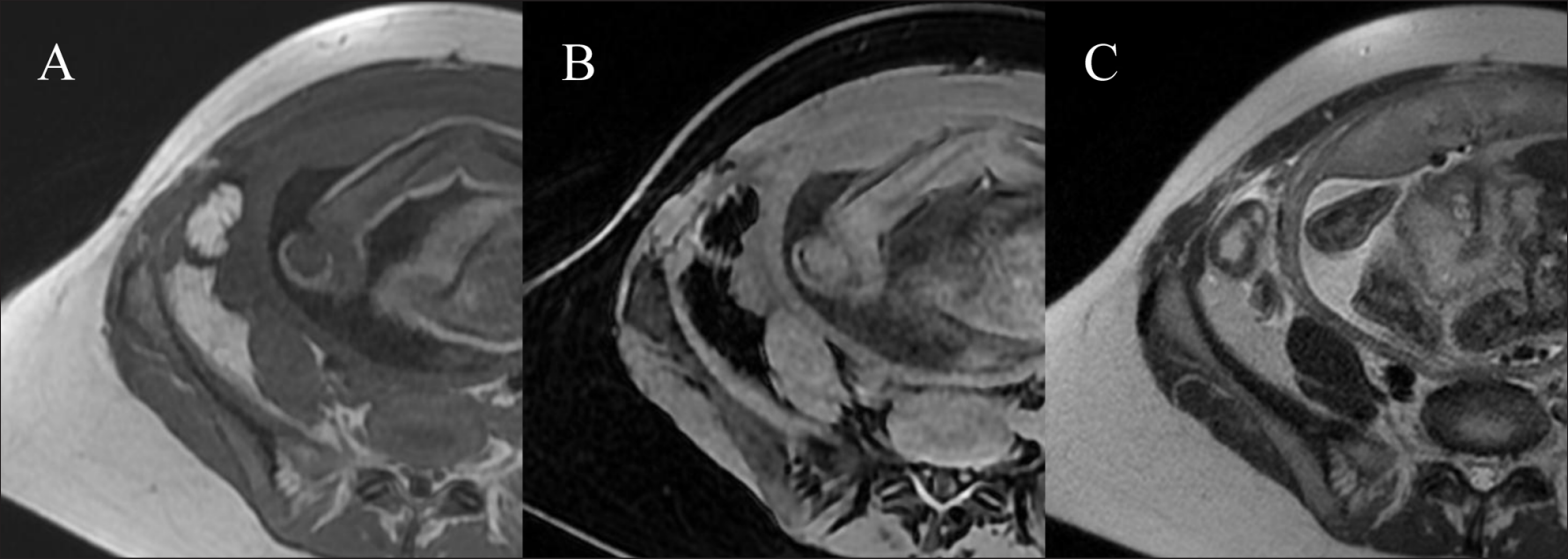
Right iliac fossa MRI. A) T1 sequence showed a anterior superficial intraperitoneal oblong mass in the right iliac fossa of 35mm hypersignal slightly less intense than normal fat with a thin peripheral hyposignal. B) T1FS sequence showed hyposignal of the mass with a fine periperal hypersignal. C) Hypersignal T2 of the mass and hypersignal T2 in the fat around.
